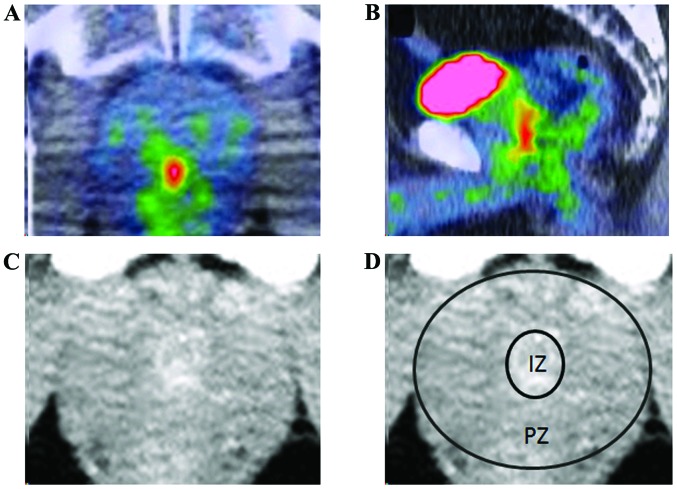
Image analysis. (A and B) Physiological uptake in the prostate urethra on coronal, sagittal and axial views was excluded. (C and D) To segment the inner zone and peripheral zone, we used the density difference in the CT portion of FDG-PET/CT. IZ, inner zone. PZ, peripheral zone.
